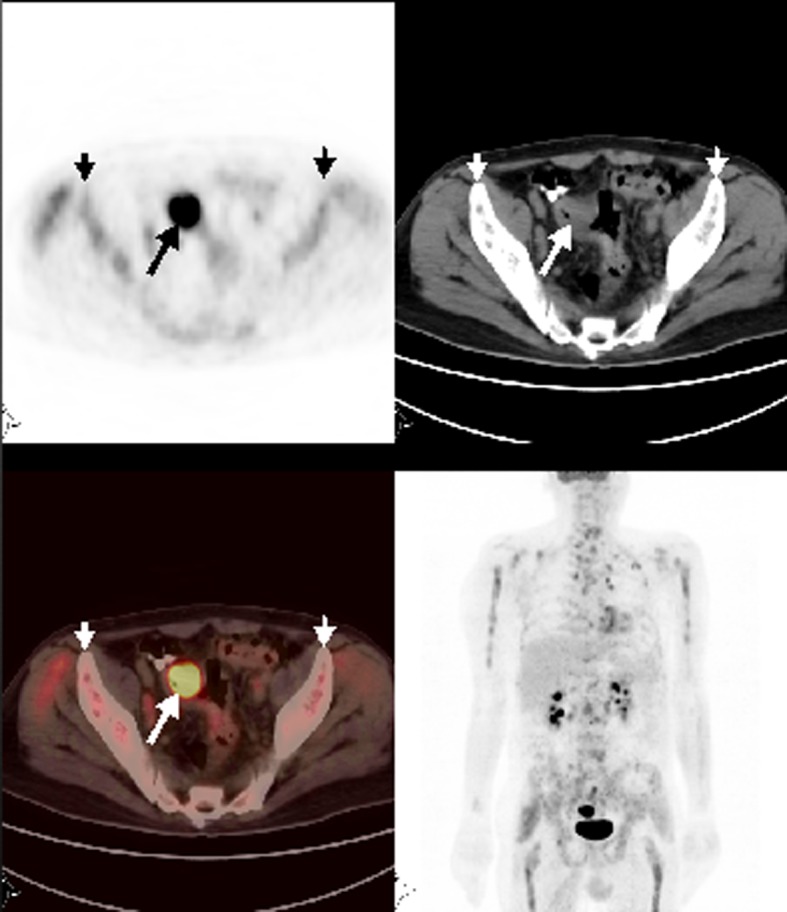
Axial and MIP PET/CT showed a mass with intense FDG uptake at the sigmoid colon consistent with primary tumor. Also, there was heterogeneously increased FDG accumulation at the skeleton suggestive for bone marrow metastases.
